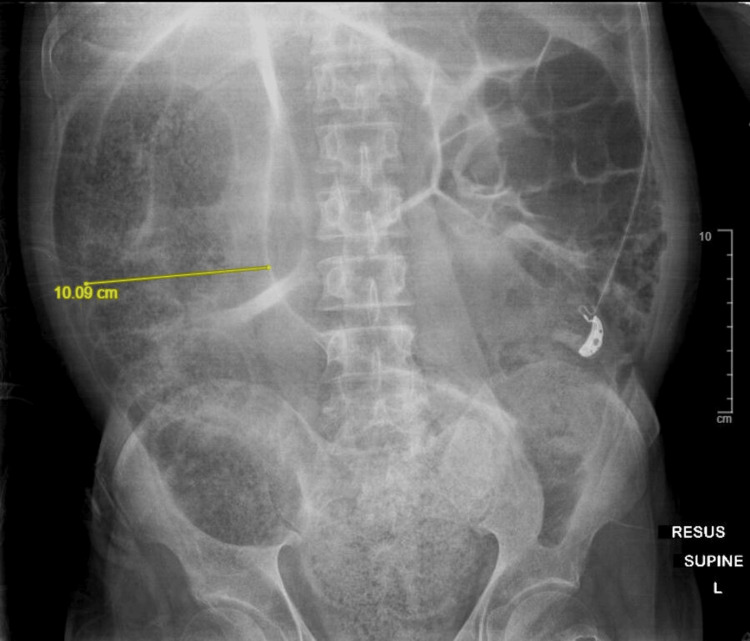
Abdominal X-ray showing faecal loading and dilated large bowel loops
